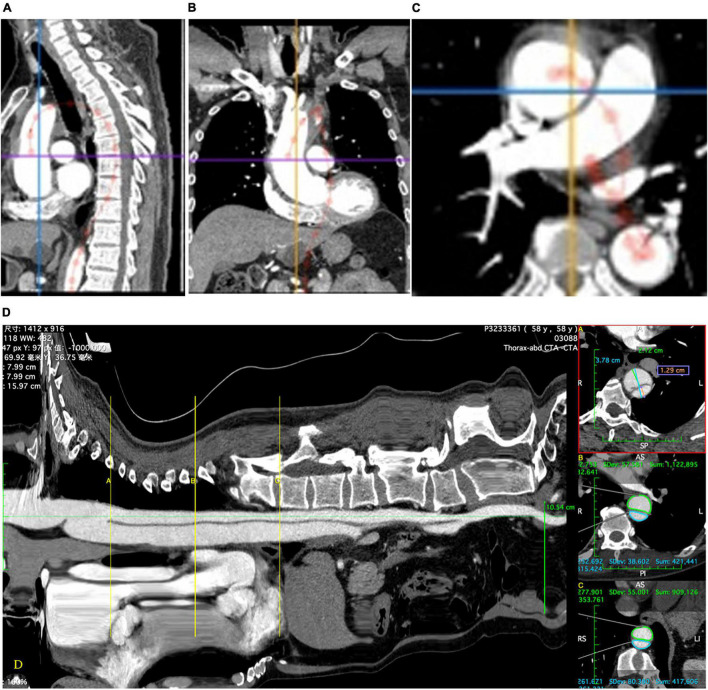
The central luminal line is installed which allows a “stretch view” to be produced (D). This allows accurate determination of anatomic landmarks and precise location of measurements. Diameters (A) and areas (B,C) are measured using multiplanar reconstructions of the computed tomographic images in the plane perpendicular to the central luminal line using the appropriate measurement tools (D).
